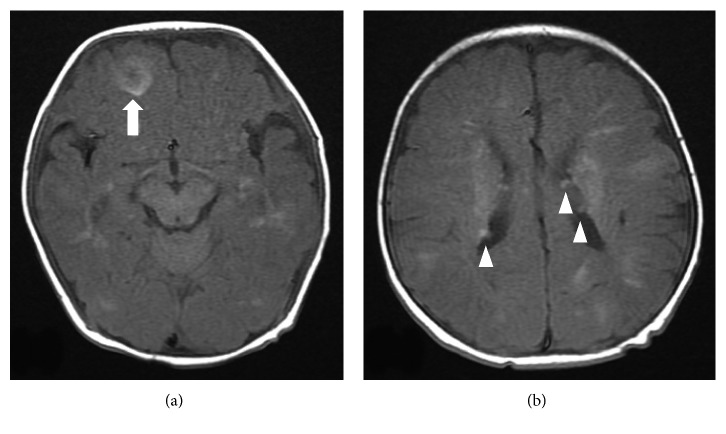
At 3 months of age, T1-weighted magnetic resonance imaging showed a right frontal cortical tuber (arrow) (a) and high signal intensity lesions in both cerebral hemispheres, with multiple nodular lesions (arrowheads) along the lateral ventricular caudothalamic grooves (b).
